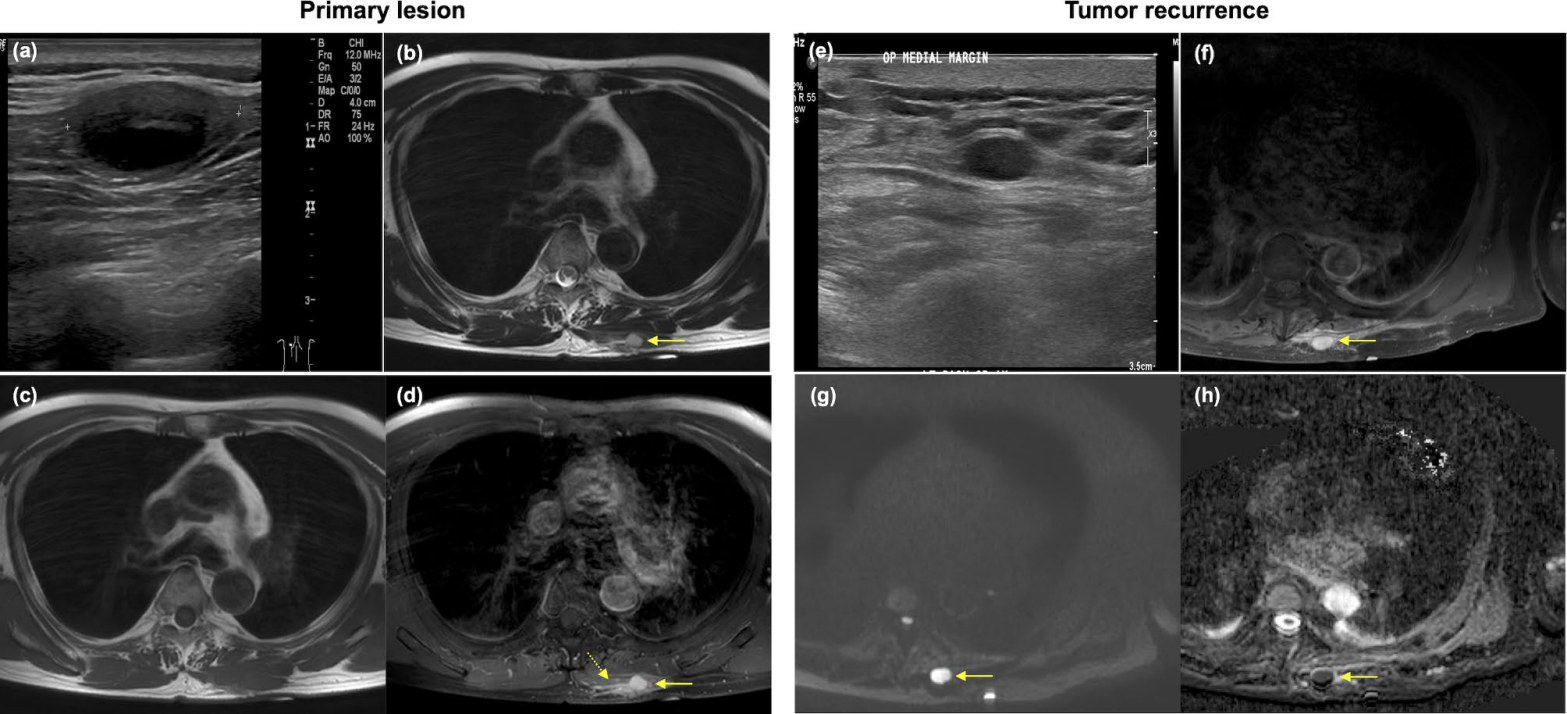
Early detection of local recurrence using short-term USG in a 67-year-old male with undifferentiated pleomorphic sarcoma. (a) Initial USG revealed a well-defined hypoechoic mass in the left trapezius muscle. (b,c,d) Axial T2-, T1-, and enhanced T1-weighted MR images show a well-defined T2 high signal intensity mass with enhancement in the left trapezius muscle, indicating a residual tumor (arrows). Ill-defined infiltration and enhancement are observed at the medial aspect of the tumor, representing postexcisional changes with or without tumor infiltration (dashed arrow). This lesion was a residual mass observed after excision at our dermatology department and pathologically confirmed as sarcoma. The patient subsequently underwent wide excision performed by an orthopedic oncologist. (e) On his 9-month postoperative USG, a newly detected 1.1-cm well-defined hypoechoic nodule was observed at the medial margin of the flap. Consequently, his MRI examination was rescheduled. (f) An enhanced T1-weighted MR image revealed a prominent enhancing mass with signal characteristics similar to those of the initial lesion. (g,h) High b-value (b = 1000 s/mm^2^) and apparent diffusion coefficient map images revealed diffusion restriction in the corresponding lesion (arrows). Wide resection was performed, and the lesion was pathologically confirmed as a recurrent tumor
